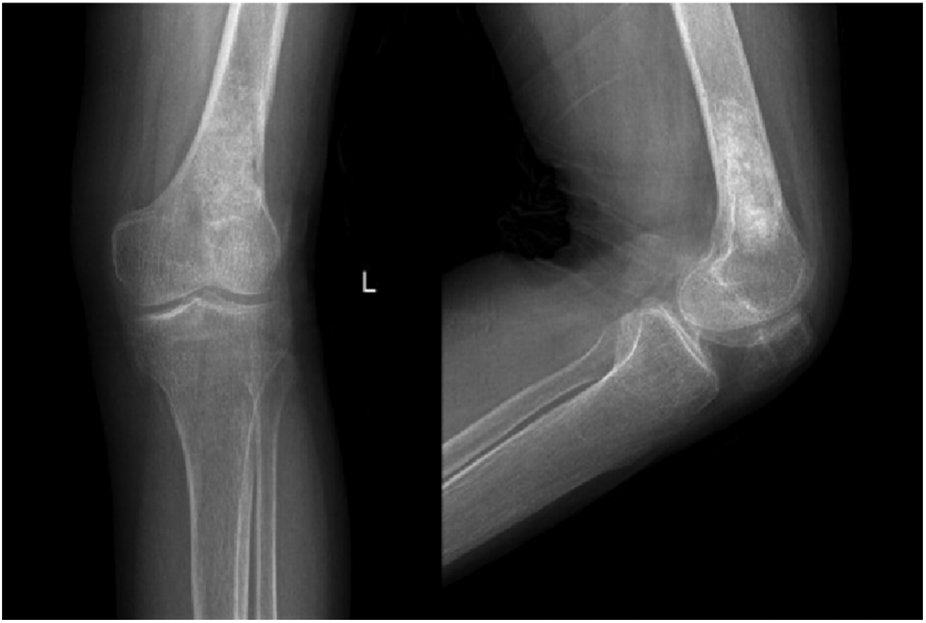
Follow-up anteroposterior and lateral radiographs of the left knee 6 months after the surgery showing good recovery of the left distal femur, consistent with postoperative changes of the left distal femur.
